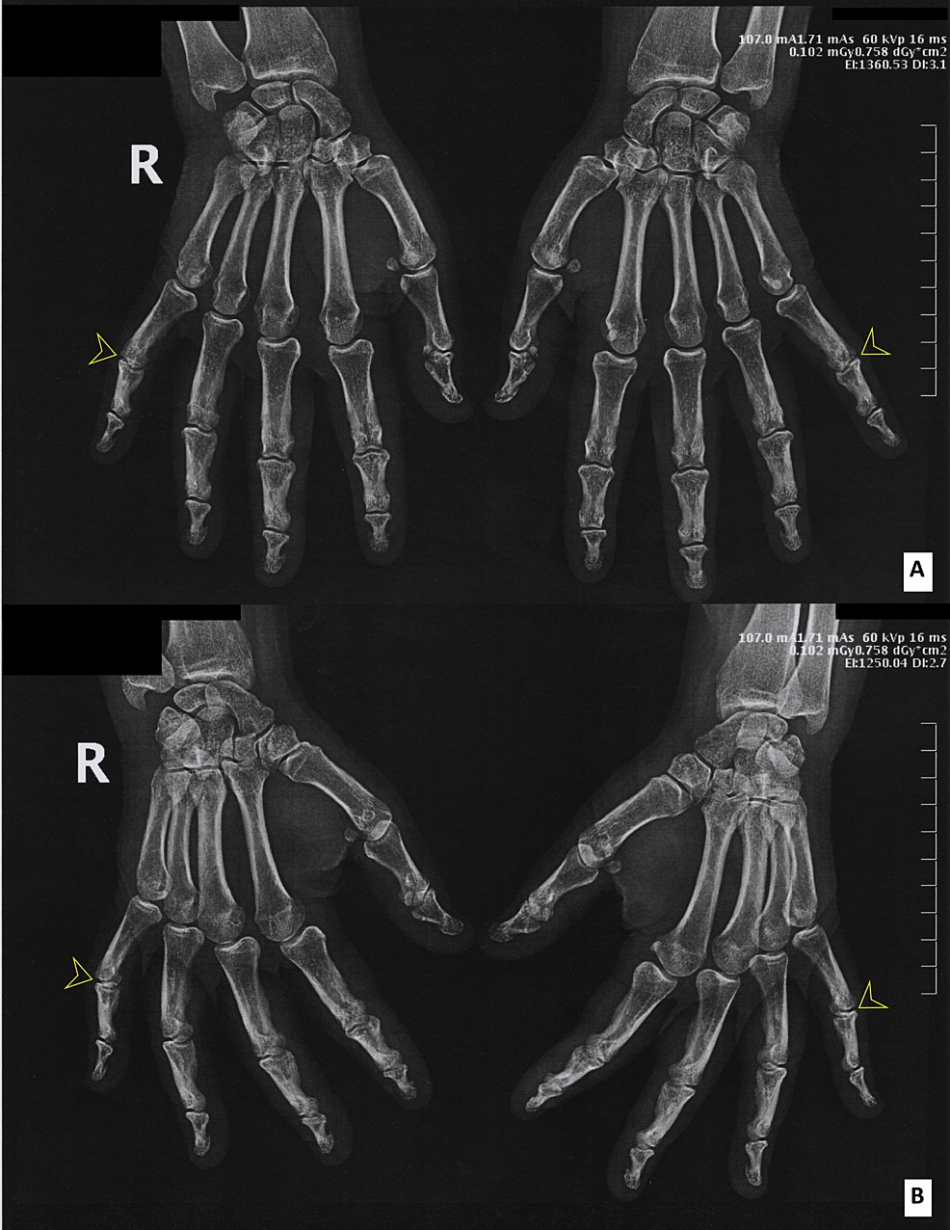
Osteoarthritis of the hands. X-ray of the hands (A: frontal view; B: oblique view): radiological evidence of bilateral incipient osteoarthritis of the proximal interphalangeal joint of the fifth finger (yellow arrowheads showing diminished intra-articular space).
